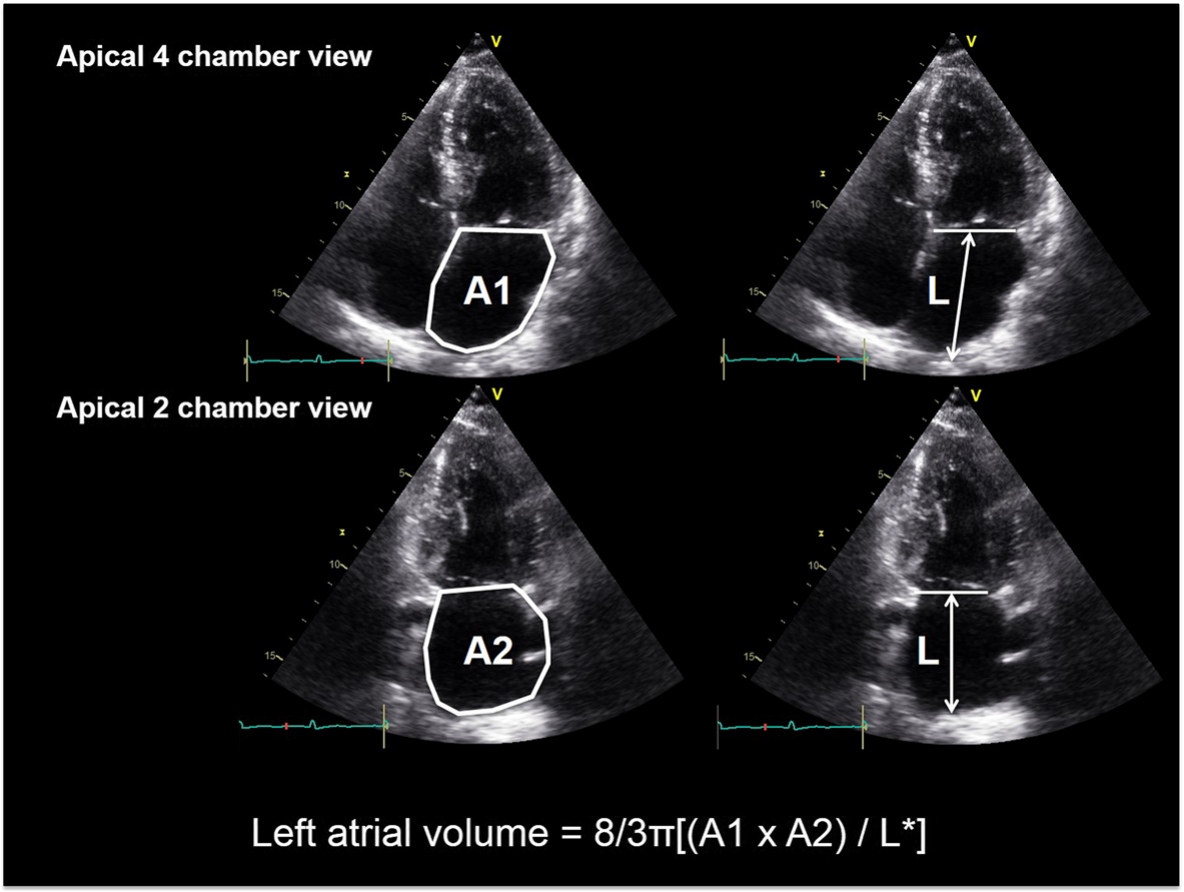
Measurement of left atrial volume from area-length method using apical 4 chamber (A1) and apical 2 chamber (A2) views at ventricular end systole. L* is the shortest of either the apical 4 chamber or apical 2 chamber length.
